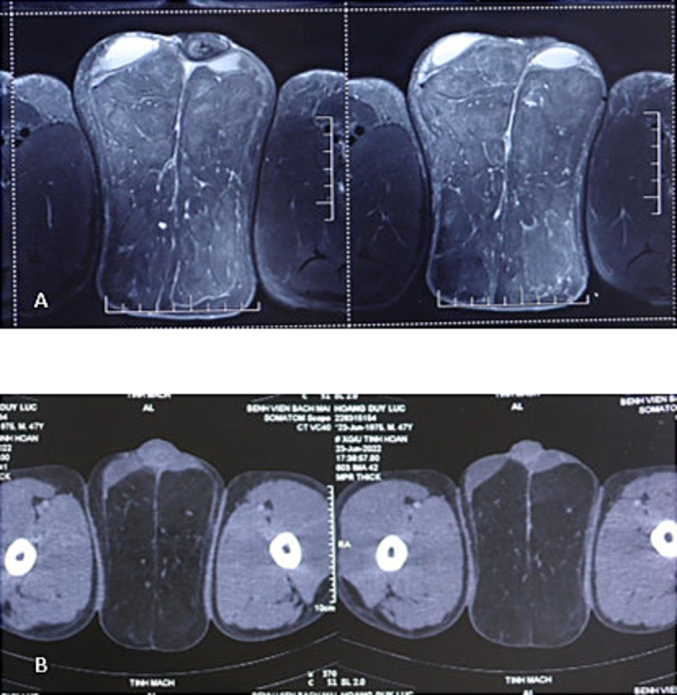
MRI images of the scrotal lesion. (A) T1- and T2-weighted coronal images showing high-signal intensity for fat deposition. The testes are pushed forwards and septae are seen. (B) T1 FS-weighted coronal images showing low-signal intensity for fat deposition.
